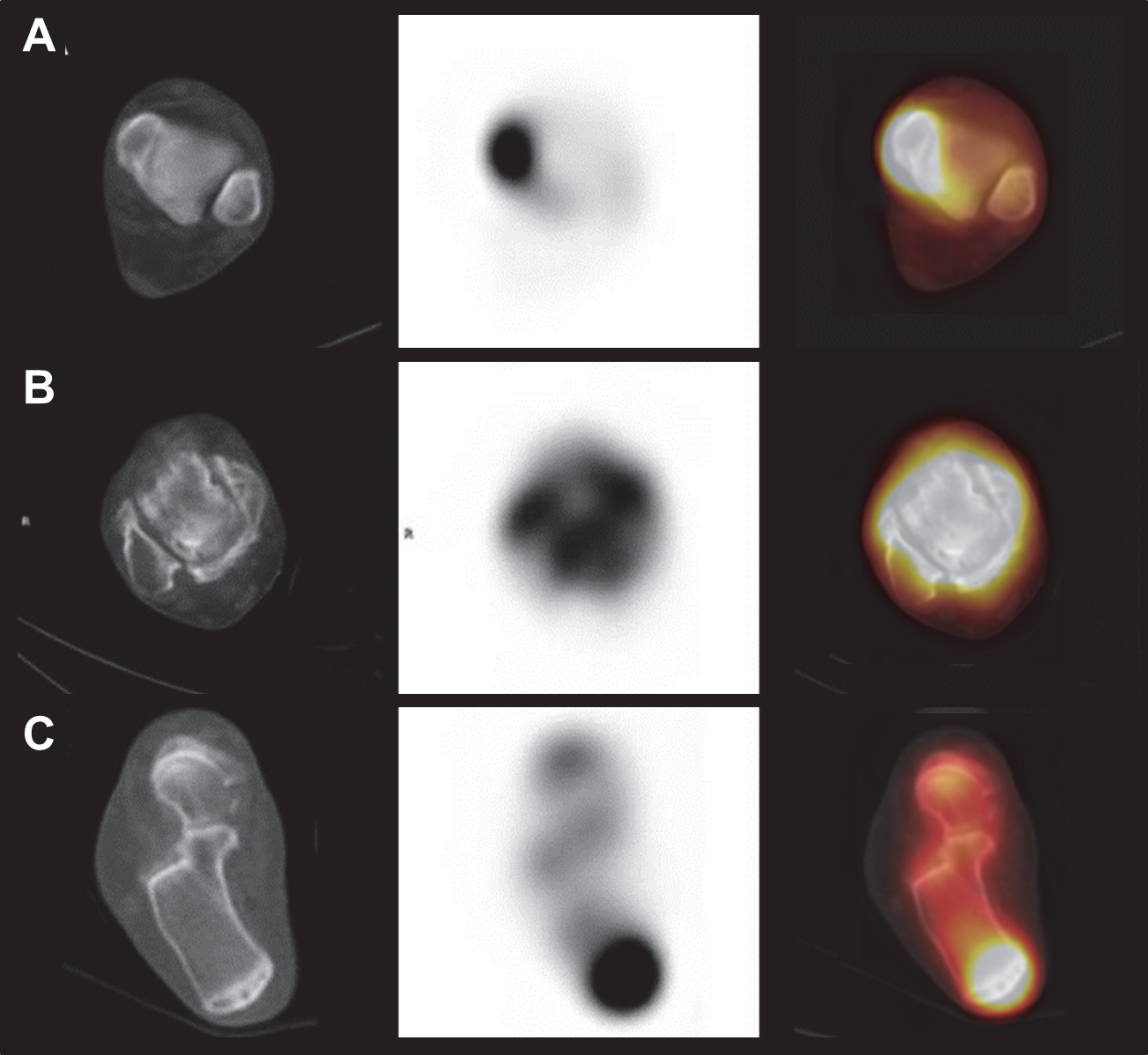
Representative images of lesion types. CT-axial (left), SPECT-axial (middle), and fusion-axial (right) images of SPECT/CT are shown; a bone lesion (contusion) involving the medial malleolus (A), a joint lesion (arthritis) involving the left talocrural joint (B), and a ligament/tendon lesion (tendinitis) involving the Achilles tendon insertion site of calcaneus (C).
